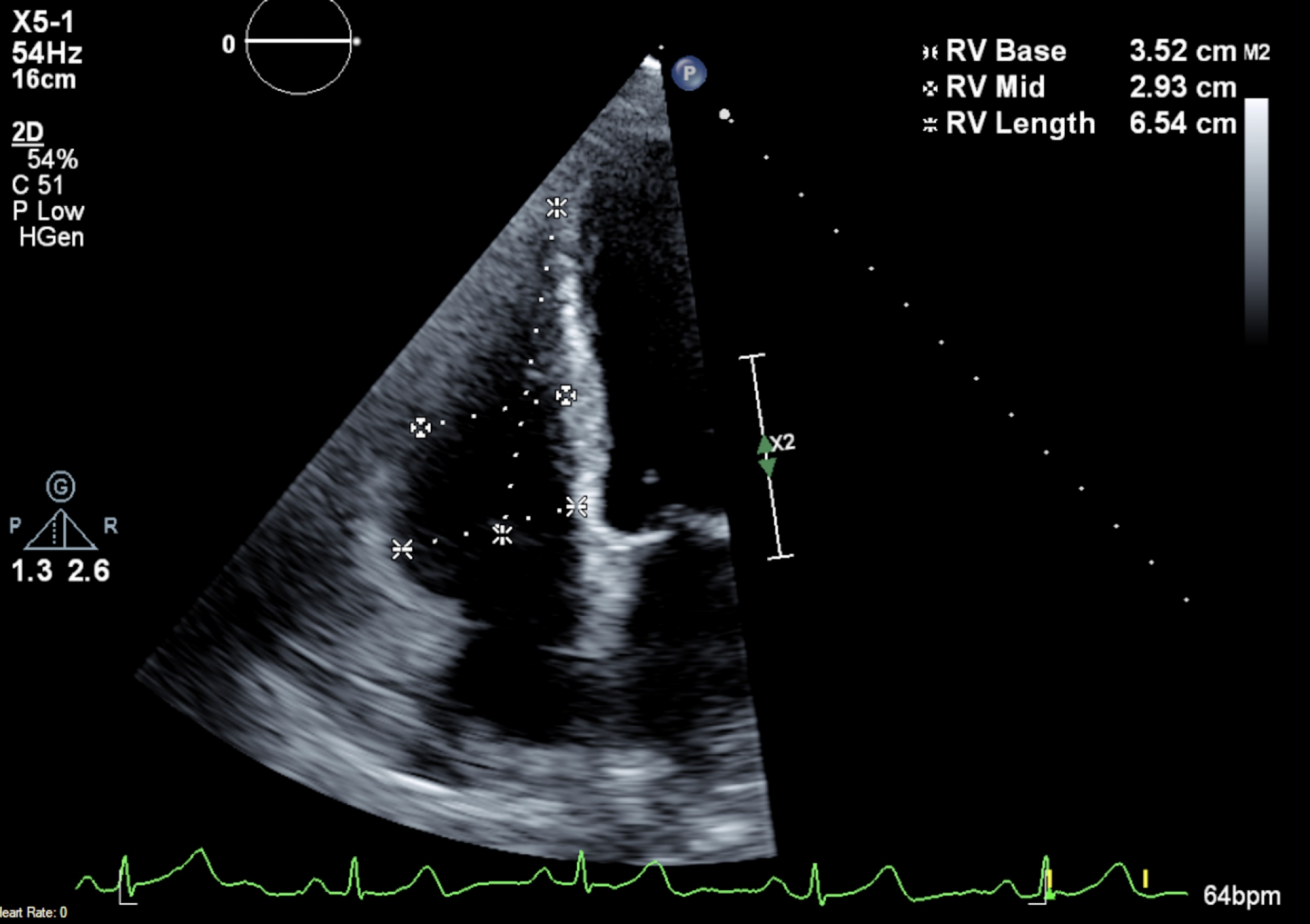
Initial TTE showing RV dimension. TTE, transthoracic echocardiogram; RV: right ventricle
